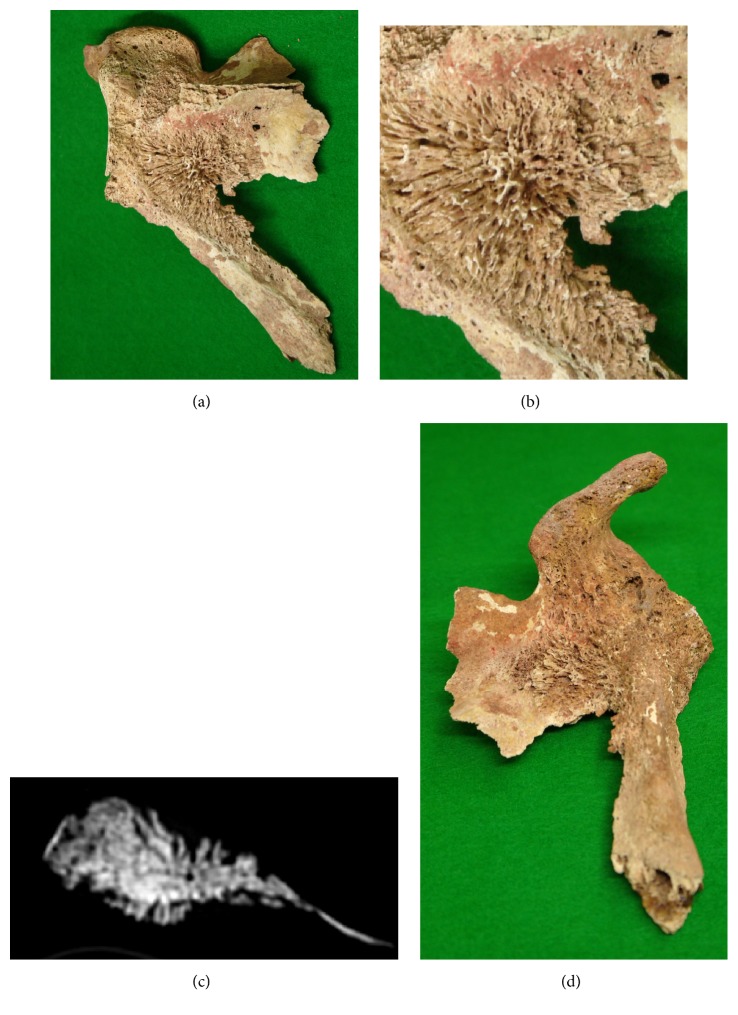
Photographs of the sunburst appearance noted on the left scapula. An osteoblastic lesion was preset, comprised of small, densely concentrating, needle-like or plate-like protruding bones expanding in a 35 × 40 mm posterior region at the base of the glenoid cavity below the base of the scapular spine (a, b). This lesion continued to the proliferative lesion on the bone surface located at the base of the coracoid (d). On CT images, the presence of needle-like osteoblastic lesions was confirmed expanding continuously across both the anterior and posterior surfaces of the base of the glenoid cavity (c).
